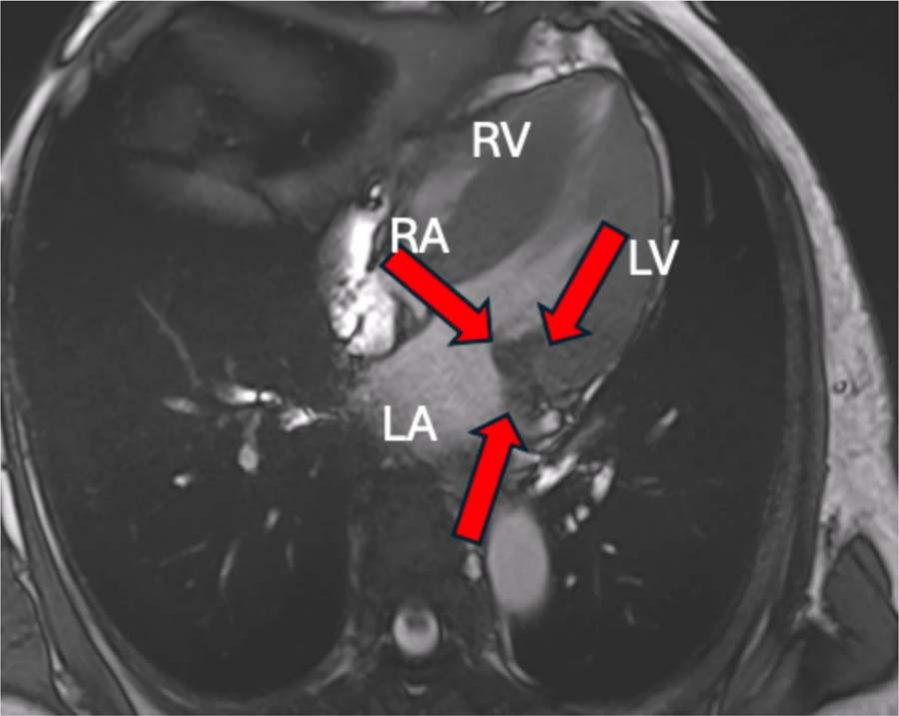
Cardiac MRI showing a hypointense mass on axial T2-weighted.
